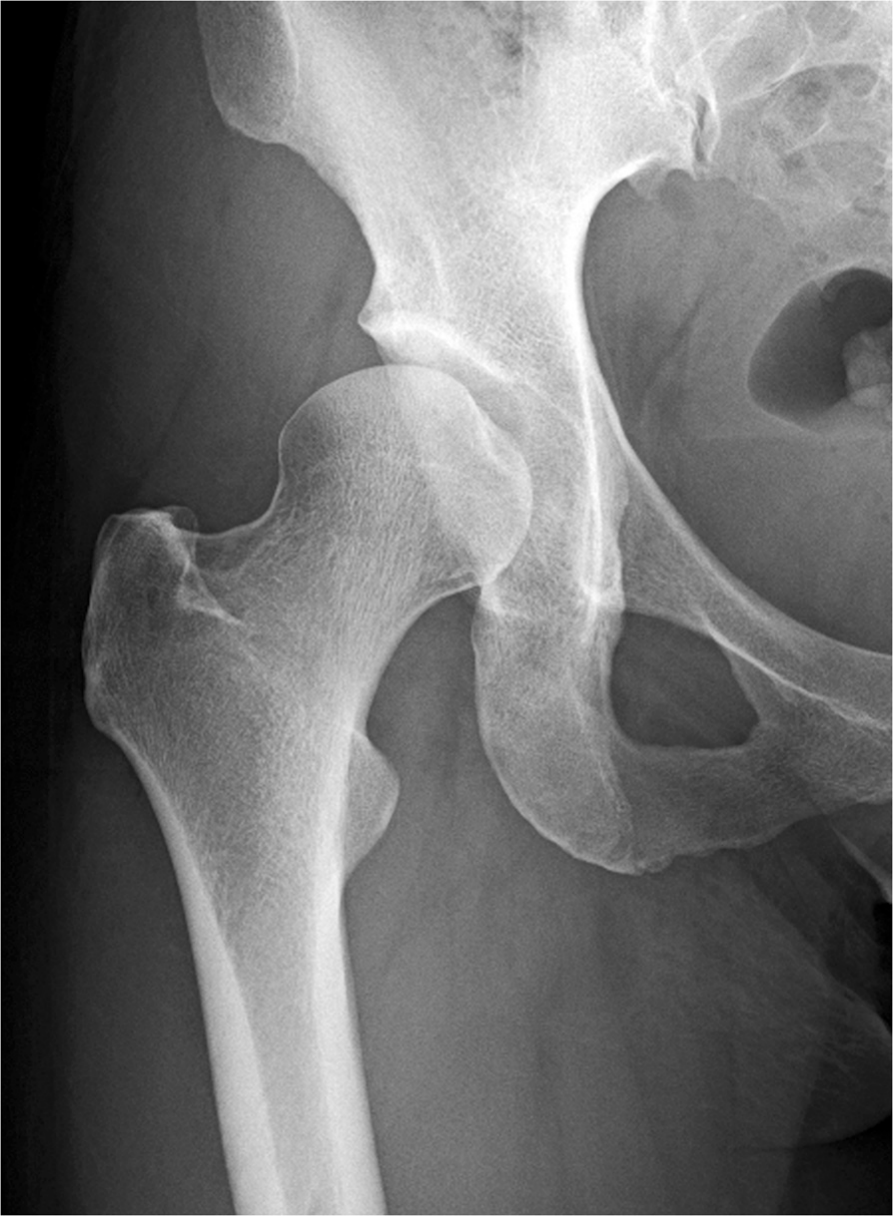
Radiographic findings of a 39-year-old woman. A preoperative radiograph showing acetabular dysplasia of the right hip.
